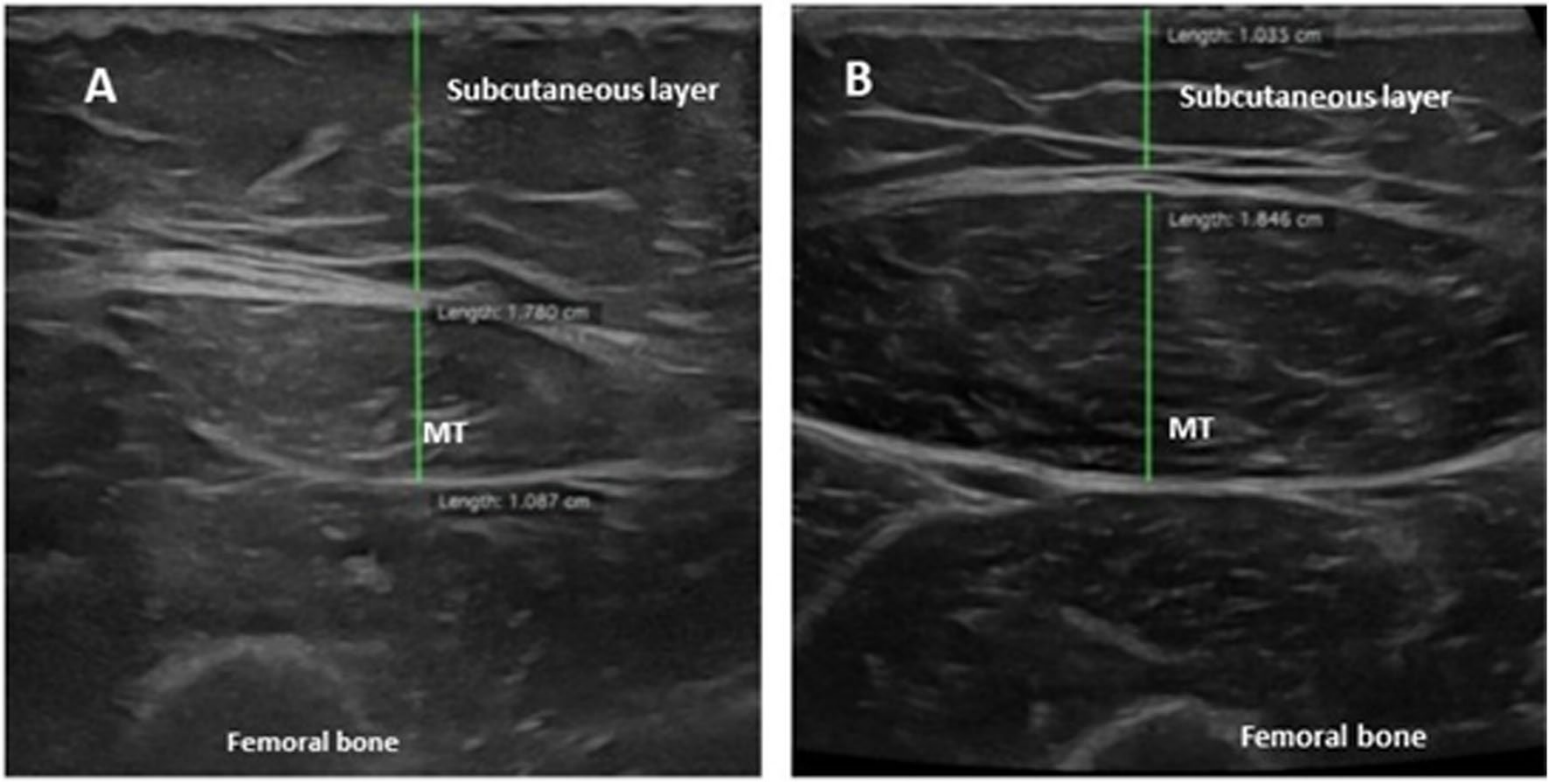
Representative ultrasound images of rectus femoris muscle in PMM2-CDG patient (A) compared to age-matched healthy subject (B). Muscle thickness (MT) is reduced in favour of increased subcutaneous tissue layer in the patient compared to age and sex matched healthy subject
